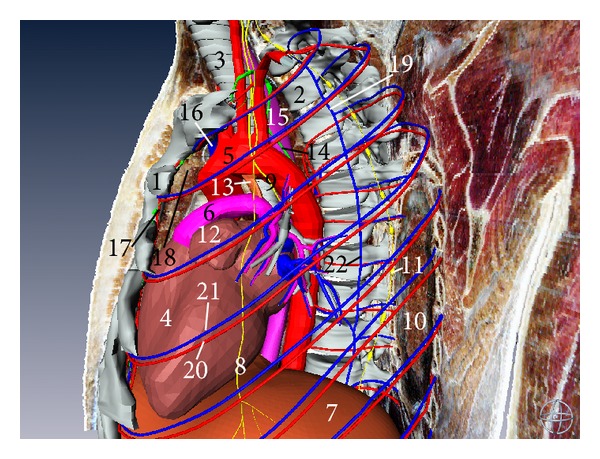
Three-dimensional model of mediastinal organs (left aspect). (1) Sternum, (2) thoracic vertebrae, (3) trachea, (4) heart, (5) aorta, (6) pulmonary artery, (7) diaphragm, (8) phrenic nerve, (9) vagus nerve, (10) left lung, (11) sympathetic trunk, (12) left auricle of the heart, (13) arterial ligament, (14) thoracic duct, (15) esophagus, (16) superior vena cava, (17) parasternal lymph nodes, (18) thymus, (19) accessory hemiazygos vein, (20) posterior intercostal artery, (21) posterior intercostal vein, and (22) hemiazygos vein.
